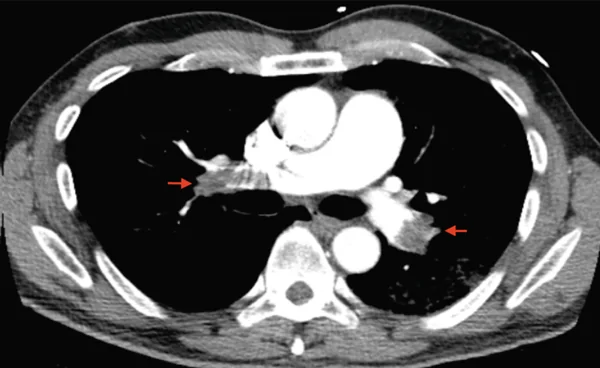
CT angiogram showing bilateral pulmonary embolism.
